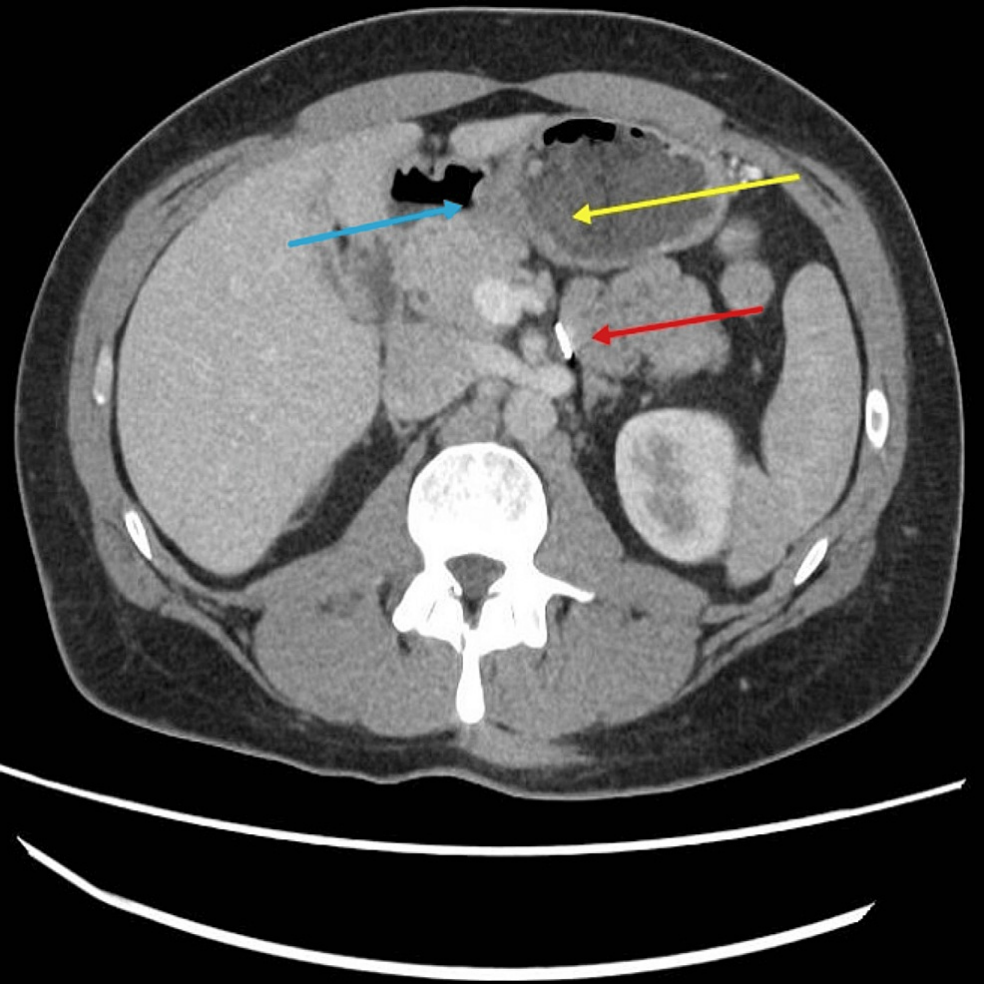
Axial view of the abdomen-pelvis computed tomogram (CT) scan showing a fluid-filled duodenum (yellow arrow) with a query lesion along the D2 (blue arrow). Additionally, surgical clips from a previously elected laparoscopic colectomy are observed (red arrow).
